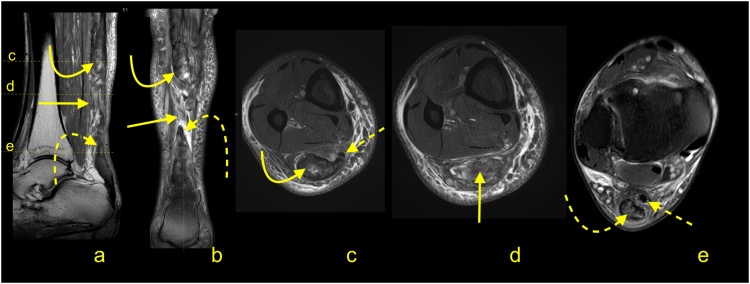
Achilles tendon rupture in midportion (straight arrow). MRI performed 4 months after the trauma (a-e). Fibrotic adhesions fill in the space between the proximal stump (curved arrow) and distal stump (dashed curved arrow). Outlines of the stumps are regular. The plantaris tendon is ruptured (dashed arrow). A- PD- weighted, b- T2- weighted with fat suppression, c, d and e- PD- weighted with fat suppression.
